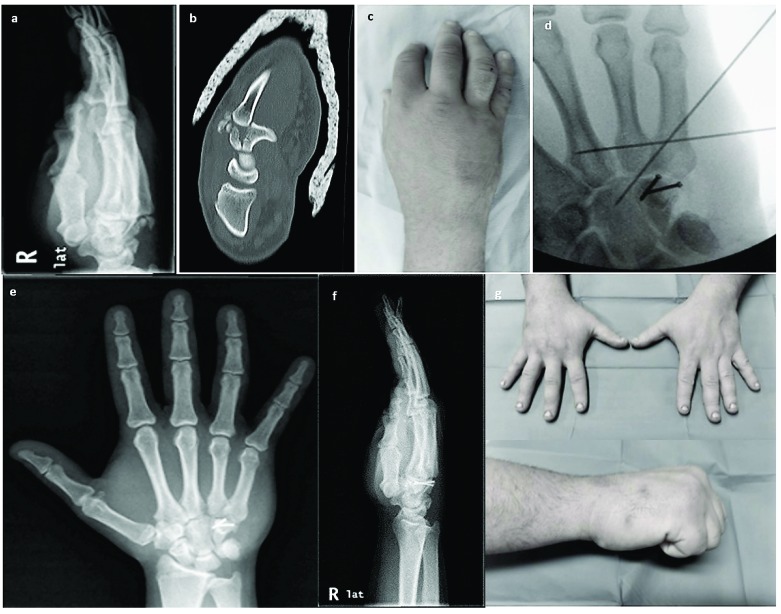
(a) Oblique 30o X-ray of the injured wrist showing dislocation of the 4th and 5th metacarpals. (b) CT scan showing the fracture of the hamate body, (c) clinical photo of the wrist showing diffuse swelling over the lesser metacarpals, (d) anteroposterior intraoperative (C-arm) X-ray showing fixation of the hamate with 2 small screws and reduction of the CMC dislocation with 2 KW, (e, f) anteroposterior and oblique X-rays of the wrist at 18 months showing healing of the hamate and congruent hamatometacarpal joint and (g) clinical photos of the wrist showing good range of motion and grip at the latest follow up.
